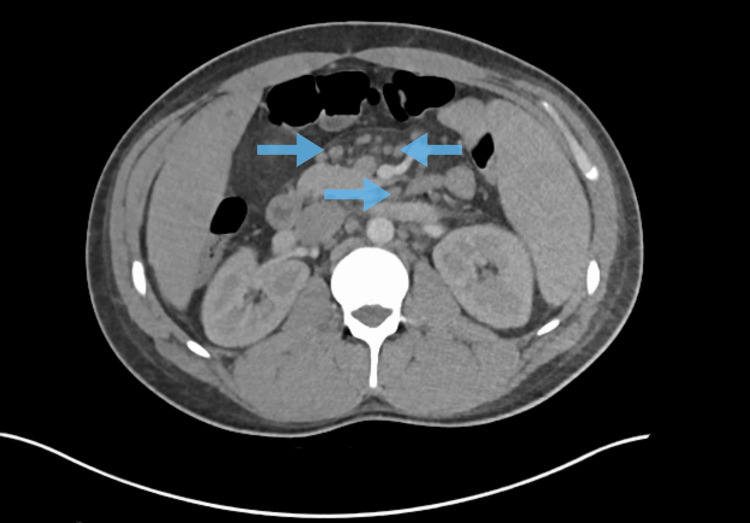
CT abdomen, axial view, showing retroperitoneal lymphadenopathy (blue arrows)
